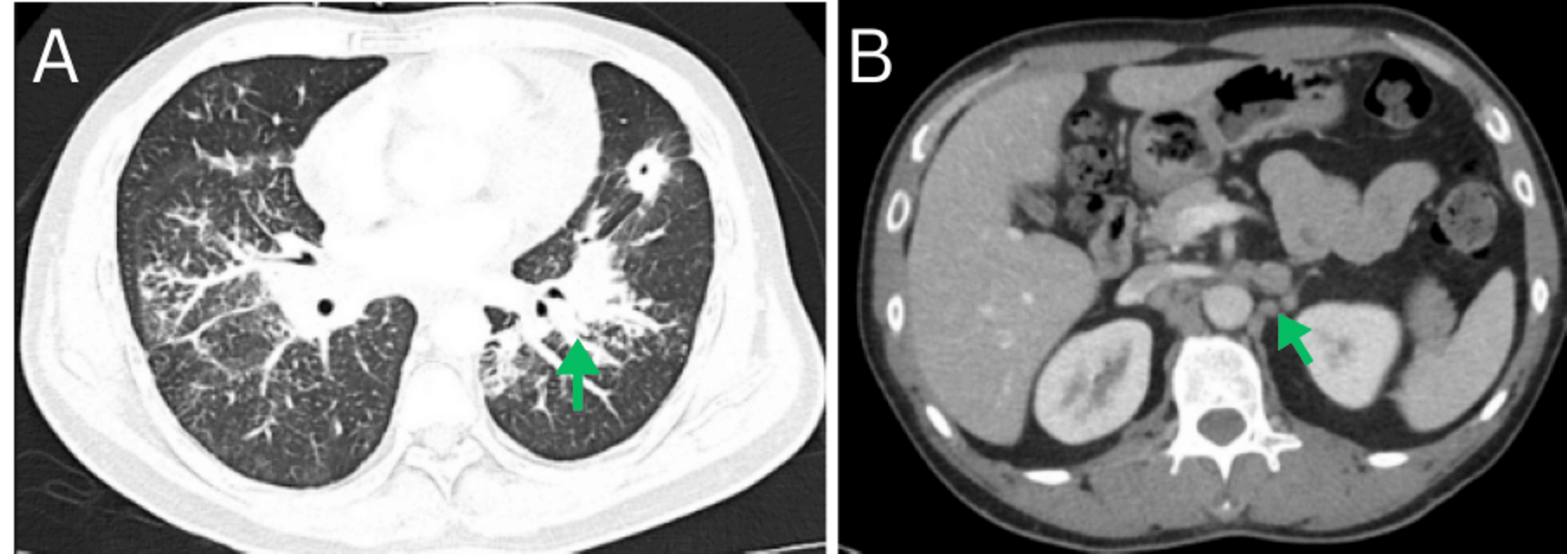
Admission chest (A) and abdominal (B) computed tomography (CT) axial sections. A: Admission chest computed tomography (CT) axial sections demonstrates a spiculated nodule with central cavitation in the left upper lobe. B: Abdominal CT reveals bilateral mediastinal and right hilar lymphadenopathy, raising suspicion for regional metastatic involvement or an underlying inflammatory condition.
